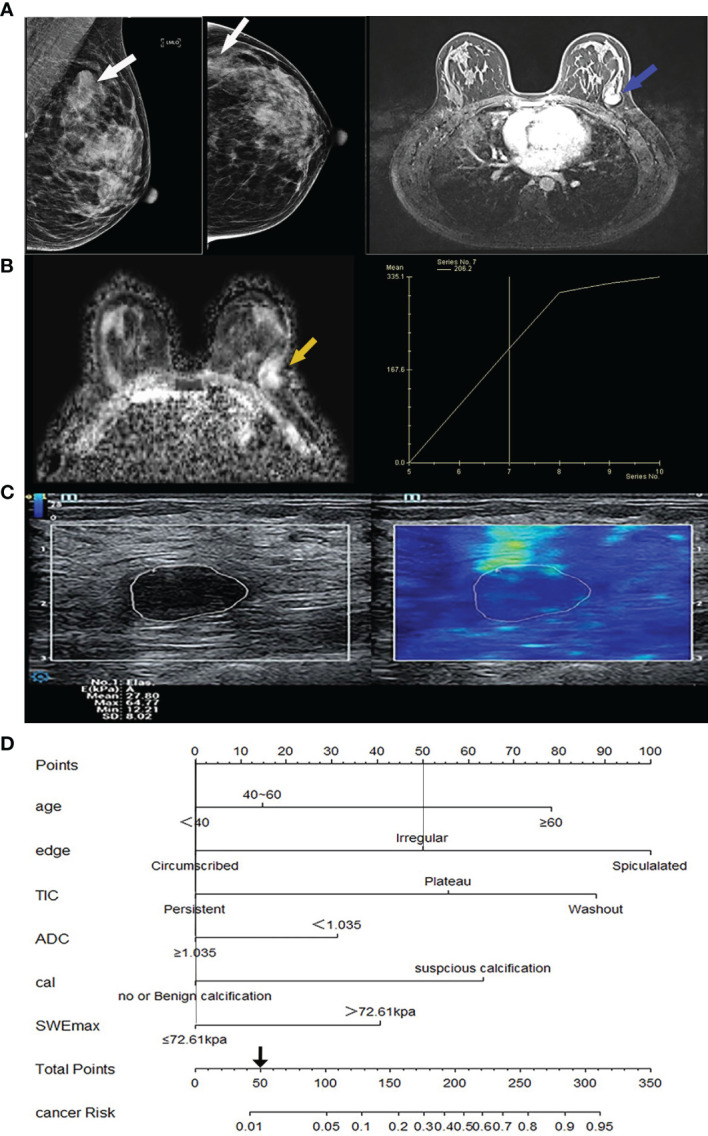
Image from a 32-year-old woman suffering from fibroadenoma BI-RADS category 4A lesions. (A) Mammography imaging on the left (lesion indicated by white arrows) and the first phase of magnetic resonance dynamic enhanced transverse axial images on the right (lesion indicated by blue arrows) showed a lobulated mass near the chest wall, without calcification. (B) Magnetic resonance transverse axial ADC image showed a high signal value of 1.4 × 10^-3^ mm^2^/s of the lesion on the left (indicated by yellow arrows) with ascending TIC curve on the right. (C) The ultrasound shear wave elastography shows SWEmax = 64.77 KPa. (D) In the comprehensive score of Nomogram, only the marginal irregularity (lobed) accounted for 50 points, which was less than 106 points, and it was downgraded into BI-RADS 3 category. cal, calcification morphology; TIC, time-signal intensity curve; ADC, apparent diffusion coefficient.
